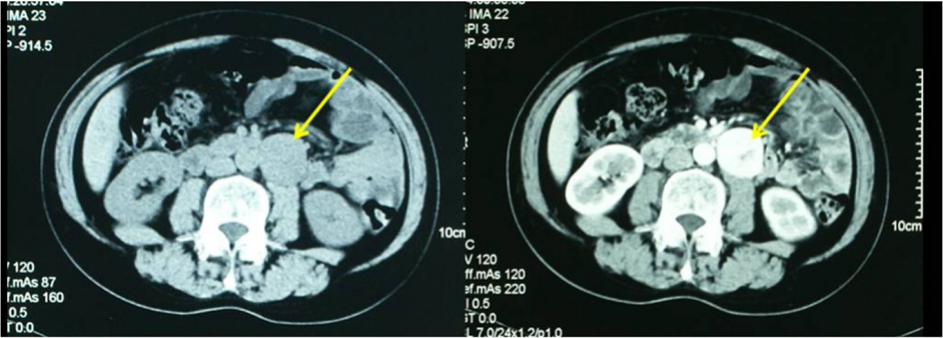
Computed tomography (CT) revealed retroperitoneal paraganglioma(yellow arrow) in the left side.
